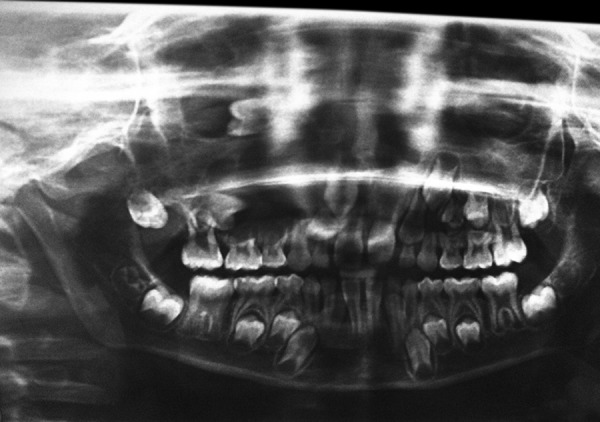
Panoramic radiograph showing cystic lesion in the right maxilla
